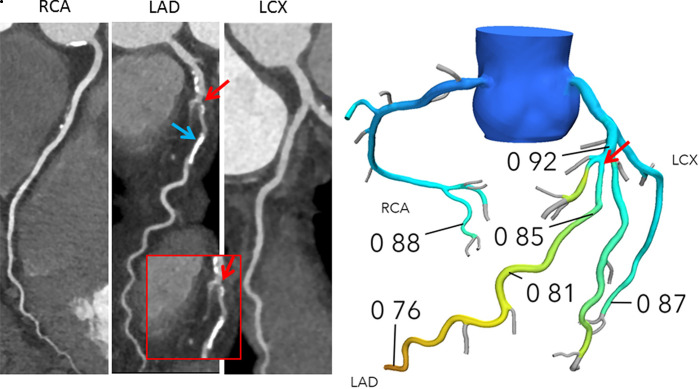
(a) Interpretation of FFR_CT_ results in a 65-year-old woman with typical angina. Agatston score, 333. Left: Coronary CT angiography curved multiplanar reconstructions demonstrate a 50%–69% proximal left anterior descending artery (LAD) stenosis (red arrow) and in the mid-LAD, nonobstructive diffuse disease. The blue arrow indicates where the lesion-specific FFR_CT_ value was assessed. Right: In the FFR_CT_ three-dimensional model, the FFR_CT_ value 16 mm distal to the stenosis was 0.85, indicating that the lesion did not cause significant pressure loss. However, FFR_CT_ was significantly low (0.76) in the terminal vessel segments. (b) Interpretation of FFR_CT_ results in a 63-year-old man with atypical angina. Agatston score, 245. Left: Coronary CT angiographic images demonstrate a 50%–69% proximal LAD stenosis (red arrow). The blue arrow indicates where the lesion-specific FFR_CT_ value was assessed. Right: In the FFR_CT_ three-dimensional model, the FFR_CT_ value 14 mm distal to the stenosis indicated that the lesion was hemodynamically significant with a value of 0.69. Coronary CT angiography and FFR_CT_ reporting are demonstrated in Figure 4. LCX = left circumflex coronary artery, RCA = right coronary artery.
